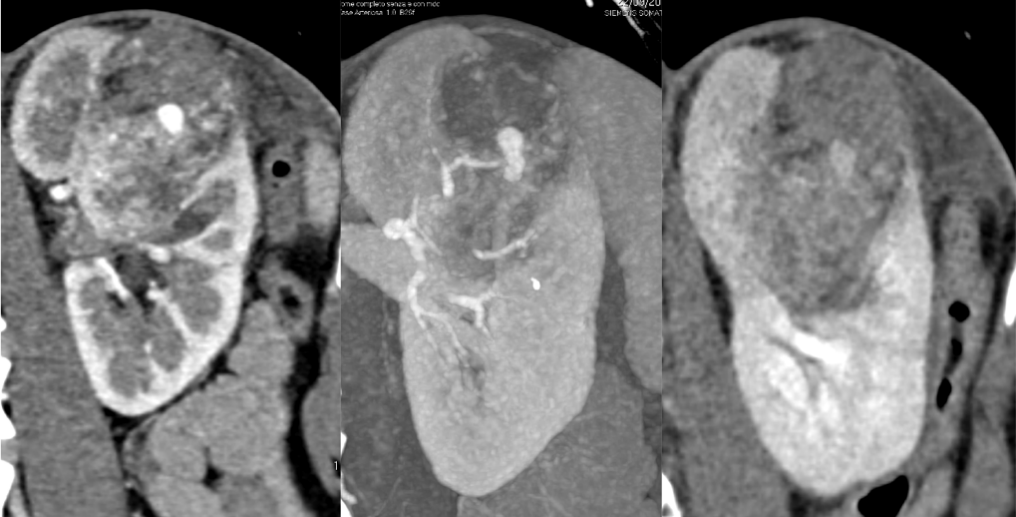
Contrast-enhanced CT scan in coronal reconstruction revealed a 4.5 × 3.5 × 7.0-cm renal AML located in the left kidney, with signs of recent blood component and cellular resentment.
